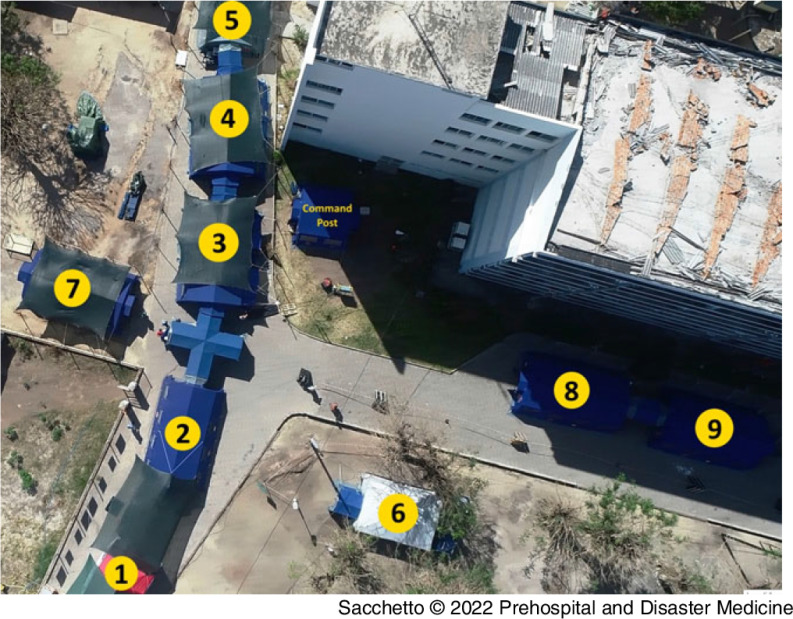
Deployed Field Hospital within the Courtyard of the Central Hospital of Beira: (1) Triage and Registration; (2) Emergency Room (ER); (3) Shock and Critical Care Room with Gynecological Corner; (4) Post-Anesthesia Care Unit and Sterilization Room; (5) Operating Theatre (OT); (6) Isolation Room for Suspected or Confirmed Infectious Disease; (7) X-Ray and Laboratory; and (8)(9) Two In-Patient Wards.
